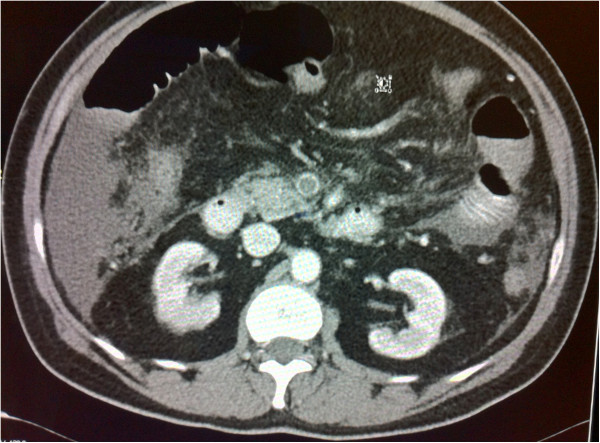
Axial computed tomography scan of the patient’s abdomen with contrast enhancement showing portal vein thrombosis causing complete occlusion of the lumen of the portal vein.
